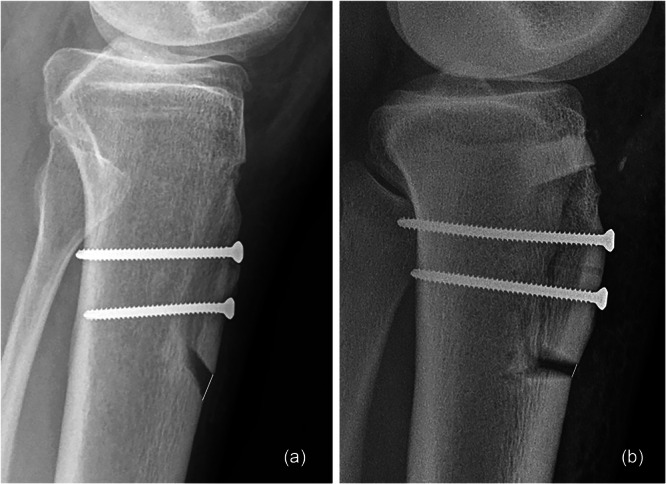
Measuring the gap postop: Sagittal radiograph measuring the gap at the anterior cortical level. (a) Dovetail distal cut with gap measurement 8.4 mm. (b) Step‐cut distal cut with gap measurement 4.1 mm.
